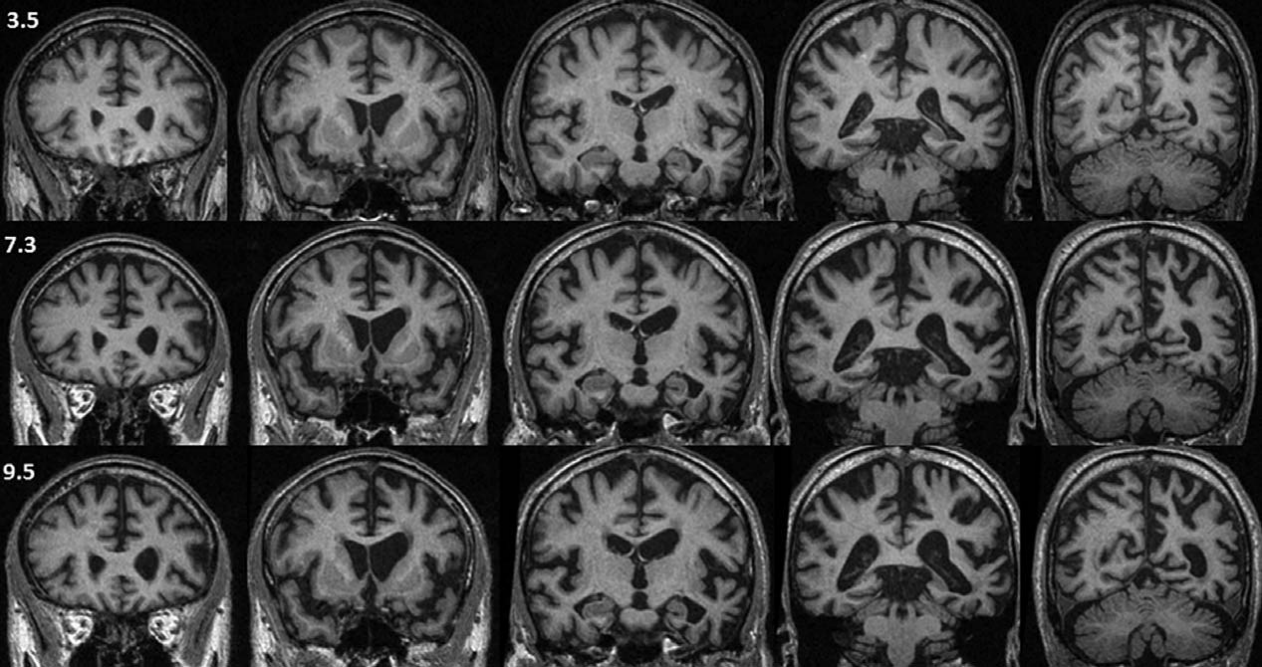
Coronal brain sections from sequential T1-weighted volumetric MRI at 3.5, 7.3, and 9.5 years from symptom onset as indicated. More anterior sections are shown on the left and more posterior sections on the right; the left hemisphere is displayed on the right in all sections.
